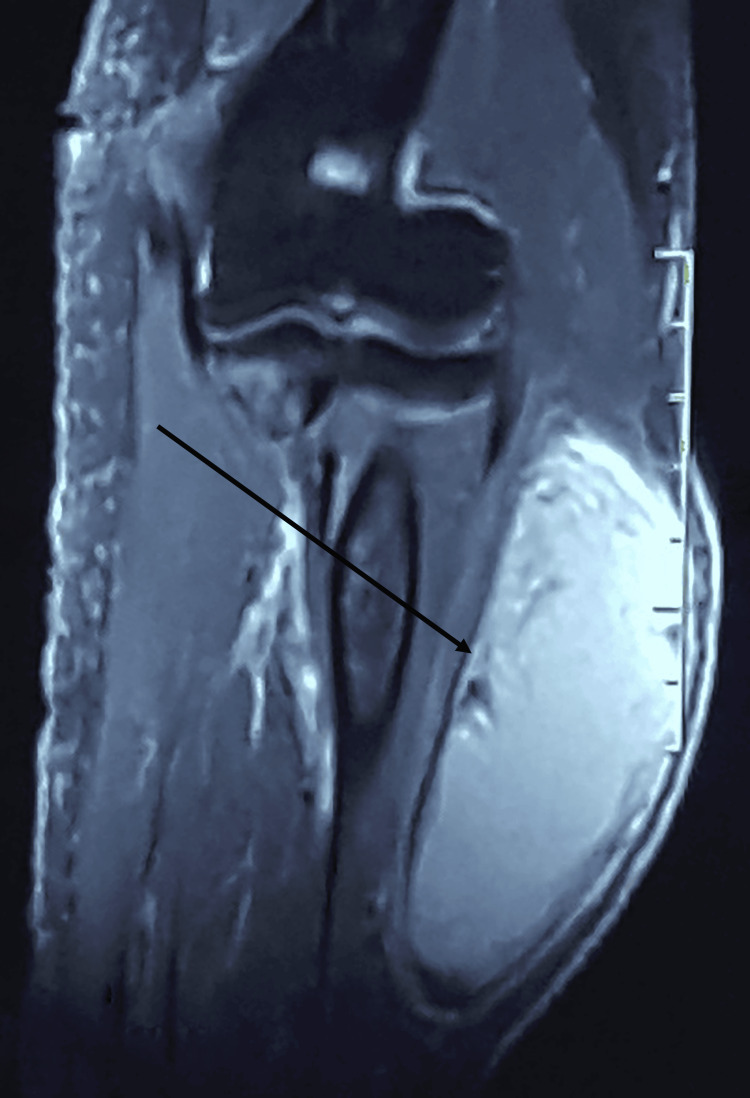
Sagittal T1 gadolinium MRI view of the right forearm
